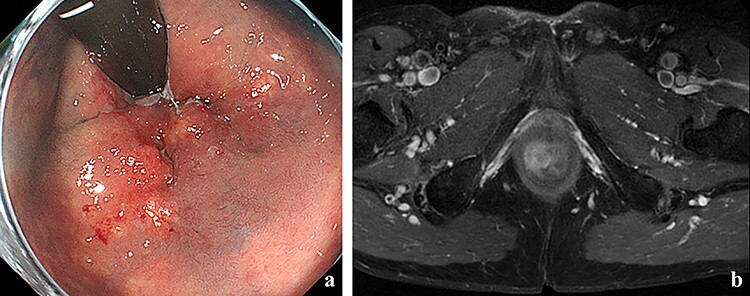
(a) Colonoscopy showed erythema of the mucous membrane from the anal canal to the rectum; (b) MRI showed that the levator ani muscle was ruptured by the tumor.
